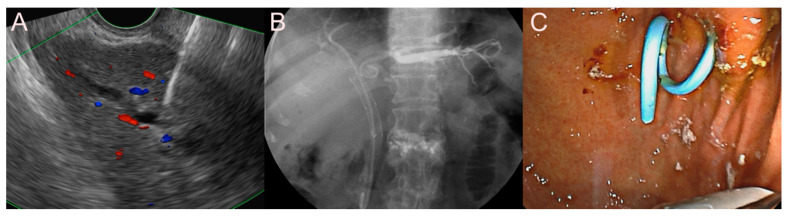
Ultrasound-guided hepatico-gastrostomy using the 7 Fr double-pigtail plastic stent (DPPS) for the obstruction of the left intrahepatic duct (IHD). (A) Endosonographic image showing a 19-gauge needle puncture into the left dilated IHD. (B) Fluoroscopic image showing the 7 Fr DPPS placed between the stomach and the left IHD. (C) Endoscopic image showing the drainage of pus-like materials through the 7 Fr DPPS.
